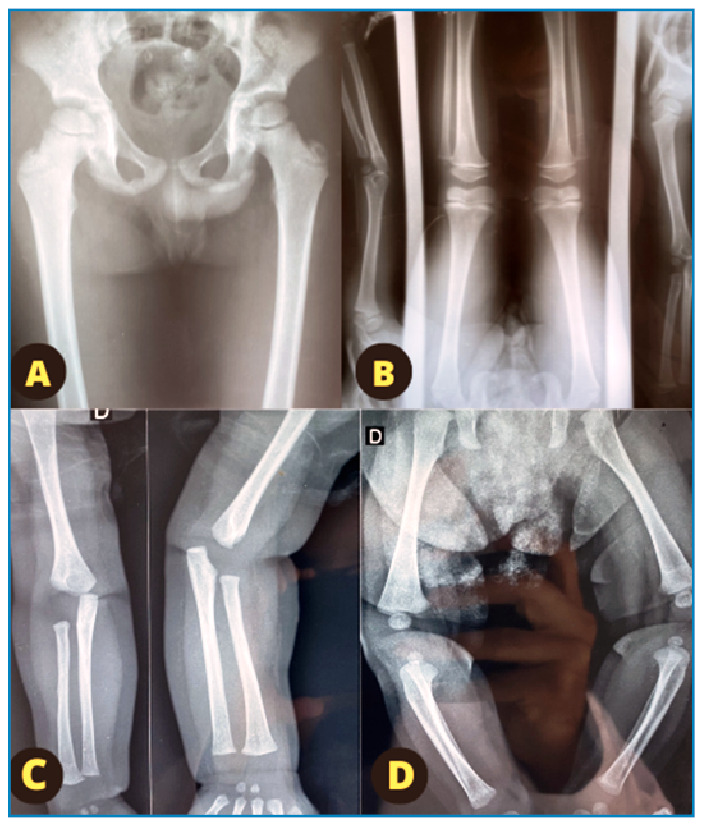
In A: leg radiograph showing no alterations in the first child after epiphyseal osteosclerosis in long bones from previous years. In B: diffuse metaphyseal alterations, suggestive of syphilis, are observed in the second child at the age of 2 years and 8 months. In C and D: metaphyseal alterations in the long bones of the third child (2 months old) are observed.
